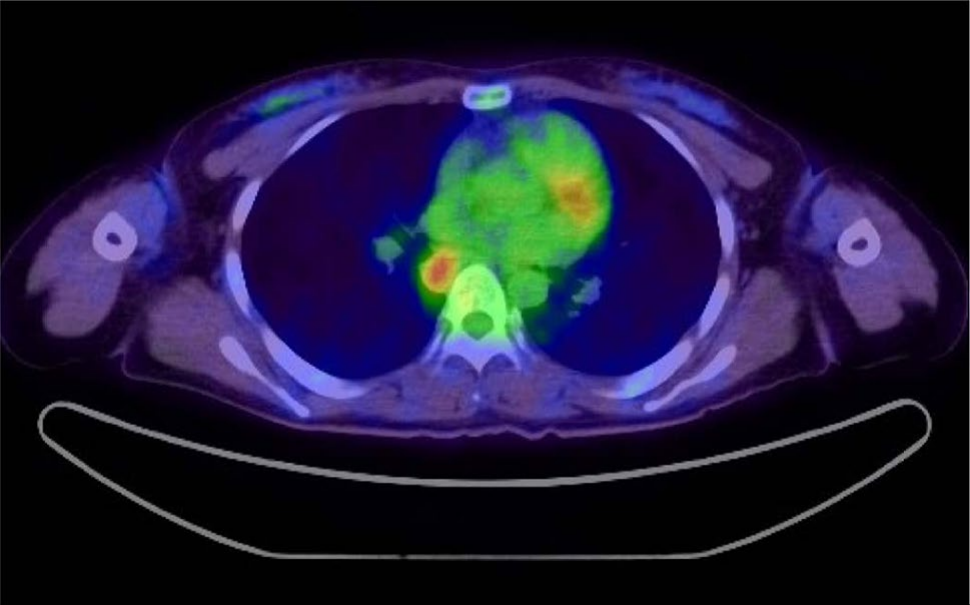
Enhanced uptake of FDG into the esophageal tumor was detected in PET-CT. *FDG* 2-deoxy-2-fluoro-18F-D-glucopyranose; *PET-CT* Positron emission tomography-computed tomography
